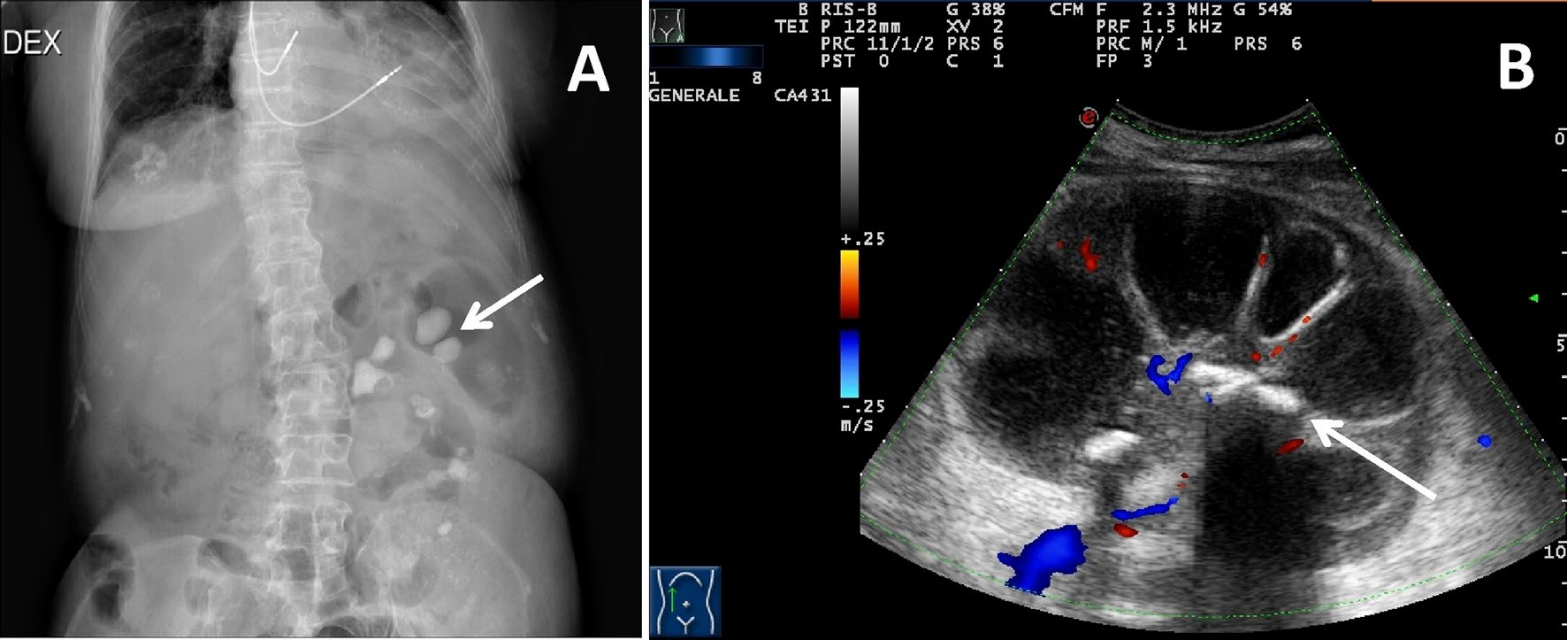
Radiographic and ultrasonographic (US) appearance of xanthogranulomatous Pyelonephritis. A 69-year-old female patient presenting with right flank pain. Conventional radiography (a) shows the presence of several big radiopaque staghorn calculus in the left kidney (arrow) and an enlargement of the renal outline. US (b) shows an enlarged left kidney with a loss of the normal renal architecture due to the cortical thinning and the presence of multiple hypoechoic areas in the parenchyma, corresponding to dilated calyxes; the renal pelvis is occupied by multiple amorphous echogenicities with acoustic shadowing corresponding to a staghorn calculus (arrow); Doppler US examination demonstrates no particular alterations in the vascularization of the organ
